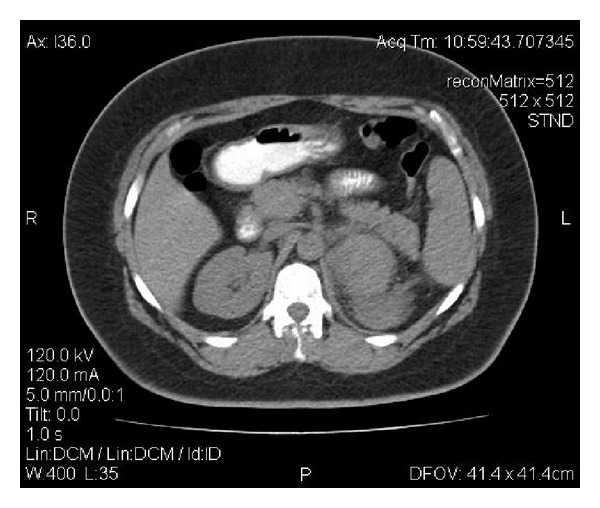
A CT scan image showing the adrenal tumor and the pertinent hematoma.
